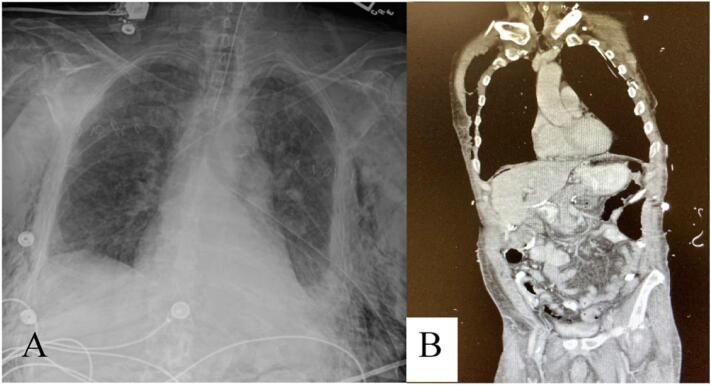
(A–B) Case presentation 2. (A) Chest X-ray HD 4, (B) Progression severe subcutaneous emphysema to the neck and abdomen.
